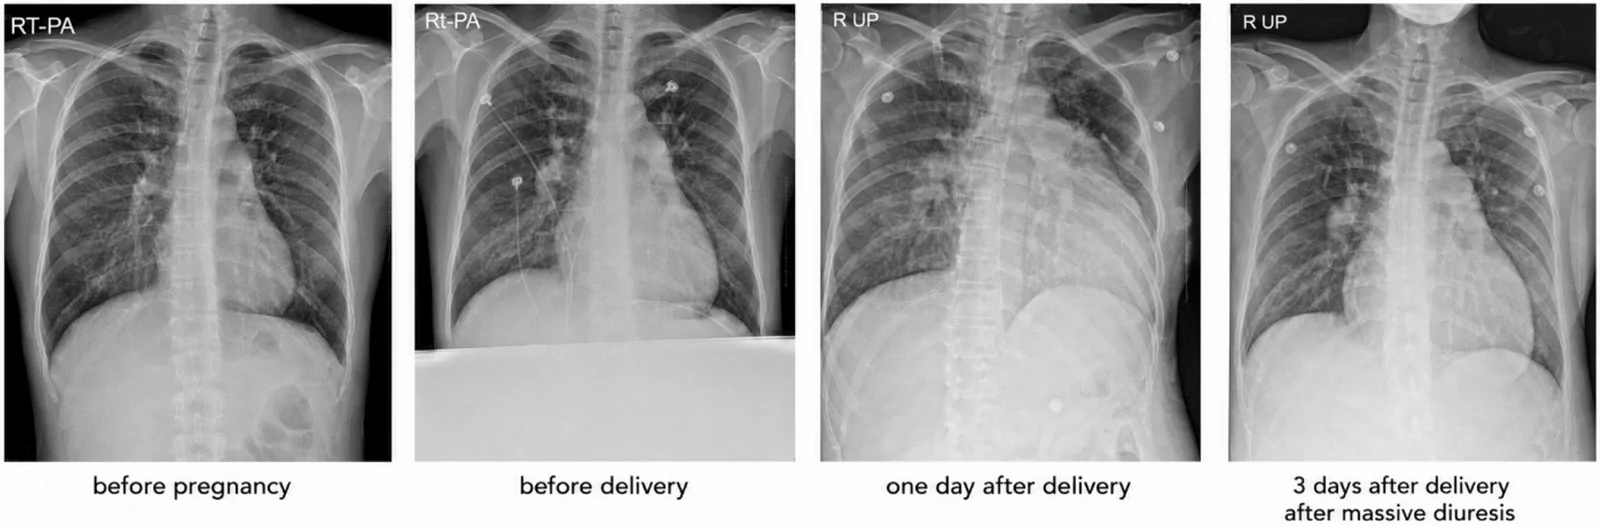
Transient cardiomegaly during the early postpartum period in women with pulmonary arterial hypertension. Serial chest radiographs from two representative patients demonstrate abrupt enlargement of the cardiac silhouette on postpartum day 1, followed by improvement by postpartum day 3, highlighting the dynamic hemodynamic burden of the immediate postpartum period
